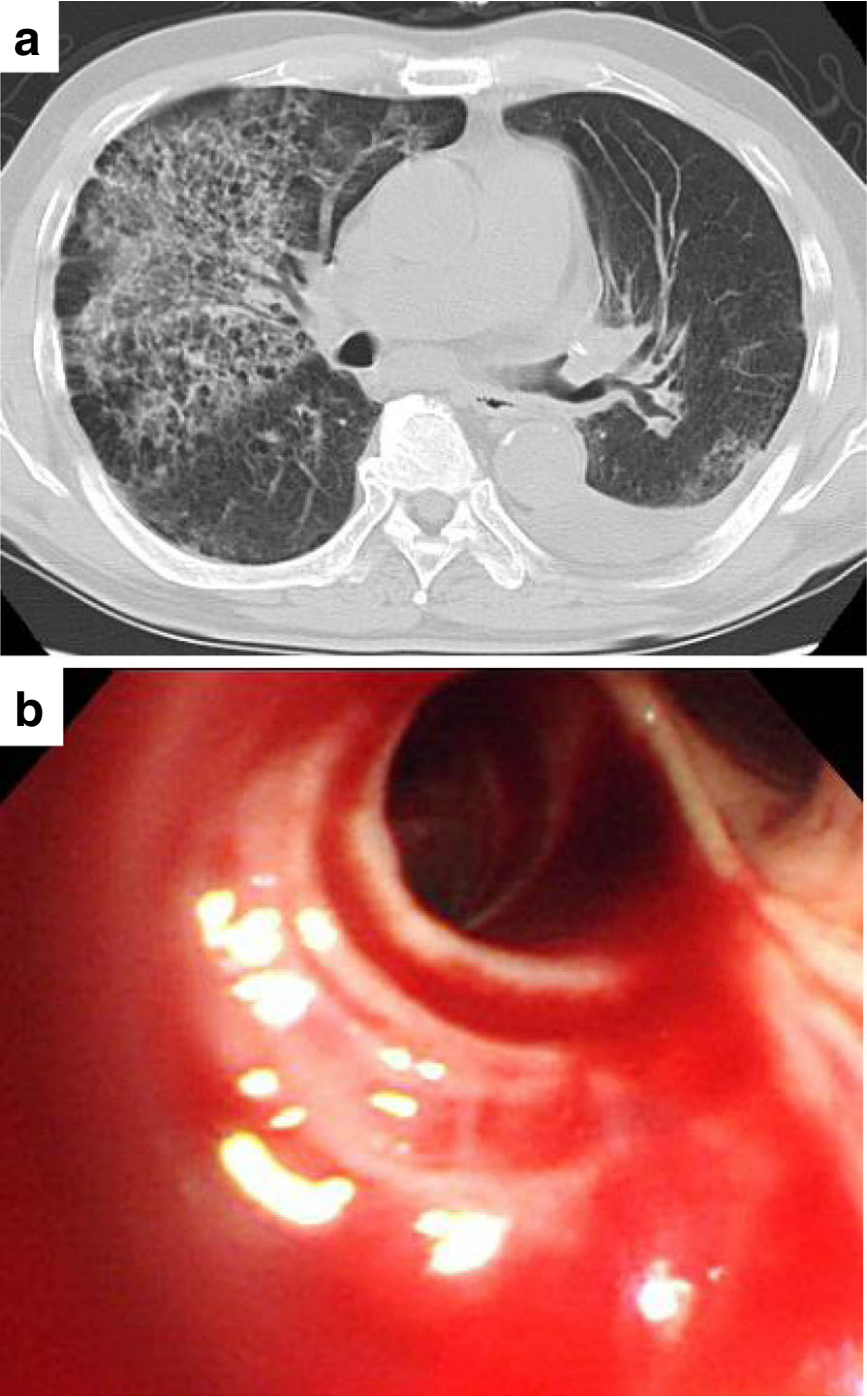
a Chest computed tomography (CT) revealed diffuse alveolar infiltrates in the both lungs. b Bronchoscopic examination revealed massive bleeding from the right upper bronchus
